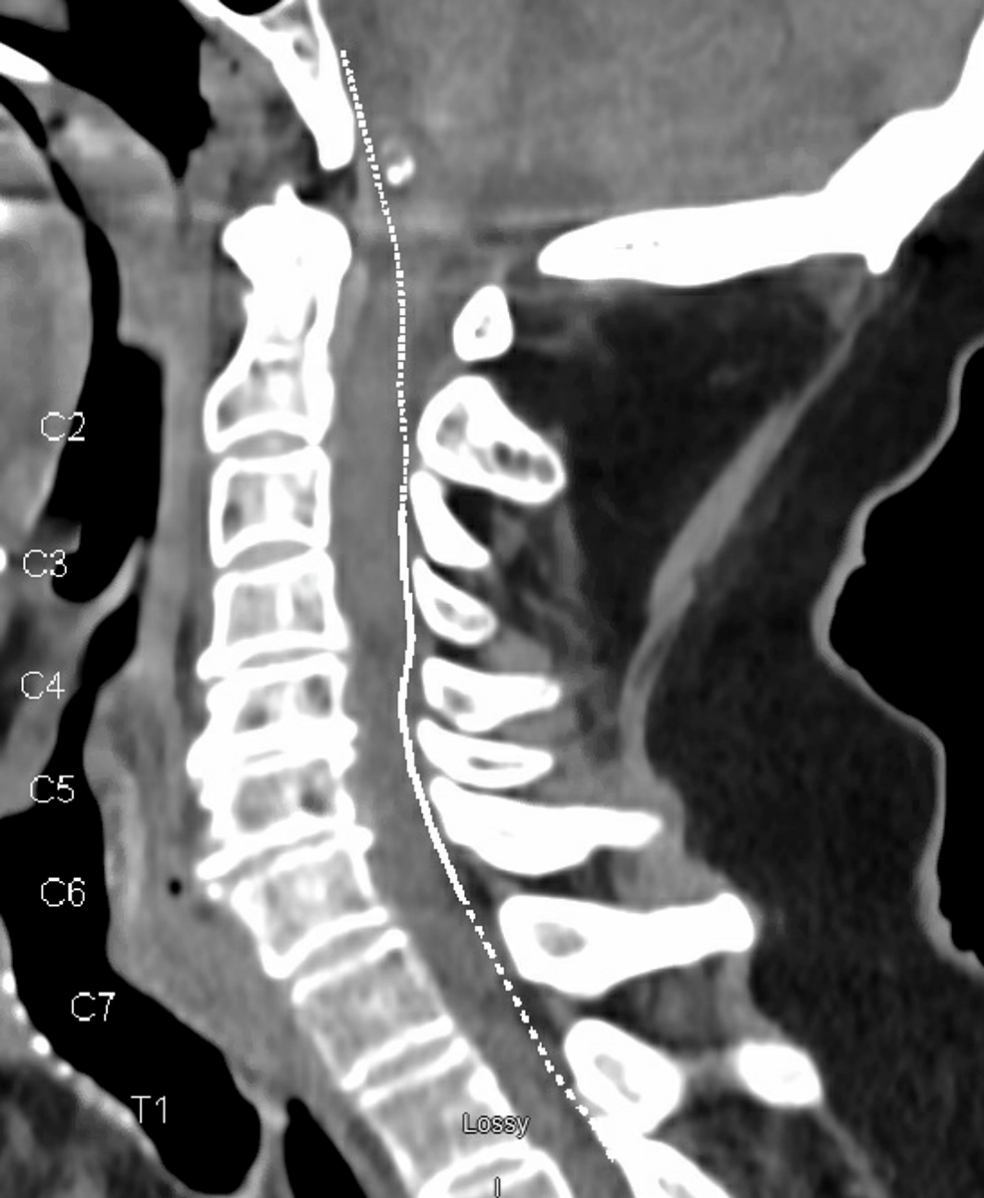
Normal CT scan of the cervical spine
